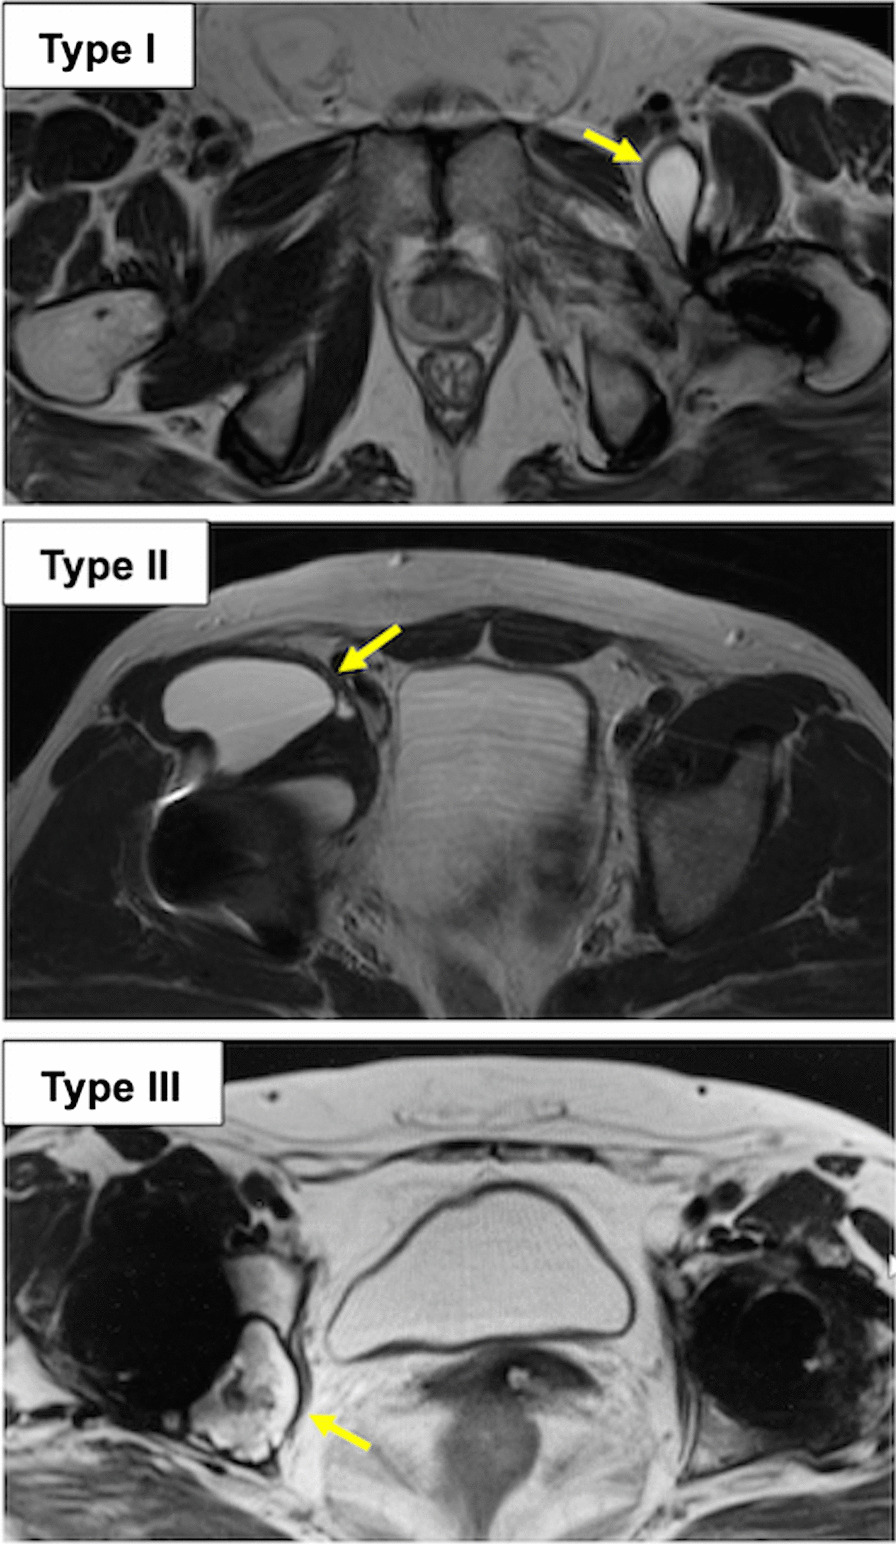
Representative metal artefact reduction sequence magnetic resonance imaging (MARS-MRI) findings of pseudotumor (arrows) classified as Hauptfleisch’s types I, II and III, respectively
